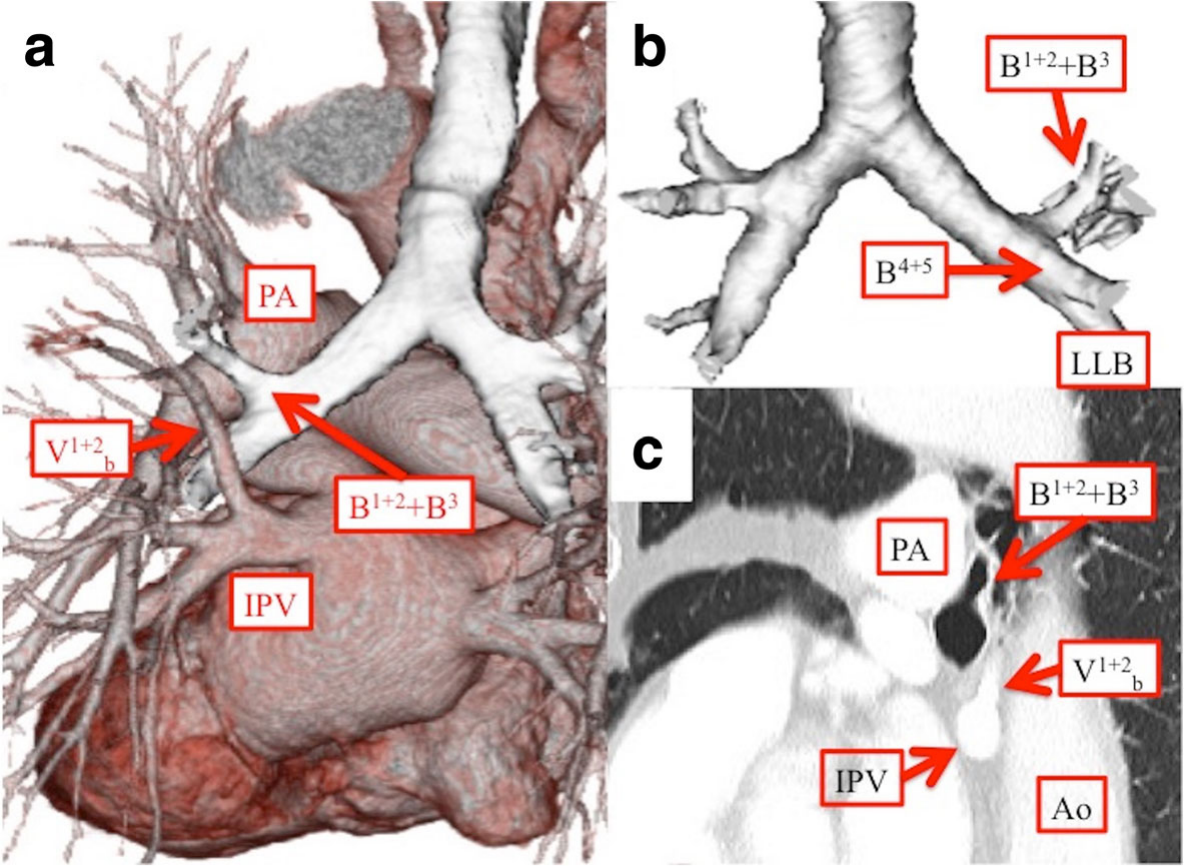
a, c Three-dimensional reconstructed image and chest CT image (sagittal) showing that the left main pulmonary artery is on the ventral side of B^1 + 2^ + B^3^ and does not cross the dorsal side of the left main bronchus and that V^1 + 2^_b_ joins the IPV on the dorsal side of the pulmonary artery. b Three-dimensional reconstructed image showing that B^1 + 2^ + B^3^ branches from the left main bronchus, followed by B^4 + 5^ and the lower lobe bronchus. Ao, aorta; IPV, inferior pulmonary vein; LLB, left lower bronchus; PA, pulmonary artery
